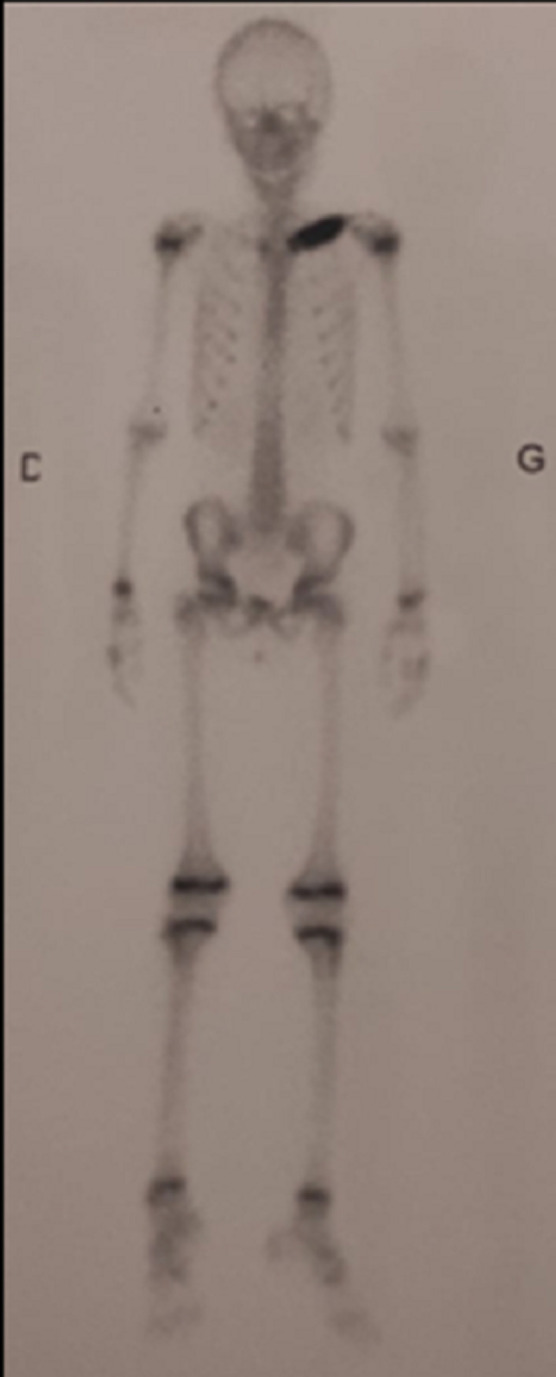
bone scintigraphy: isolated isotop uptaking on the inner part of the left clavicle with no other location
